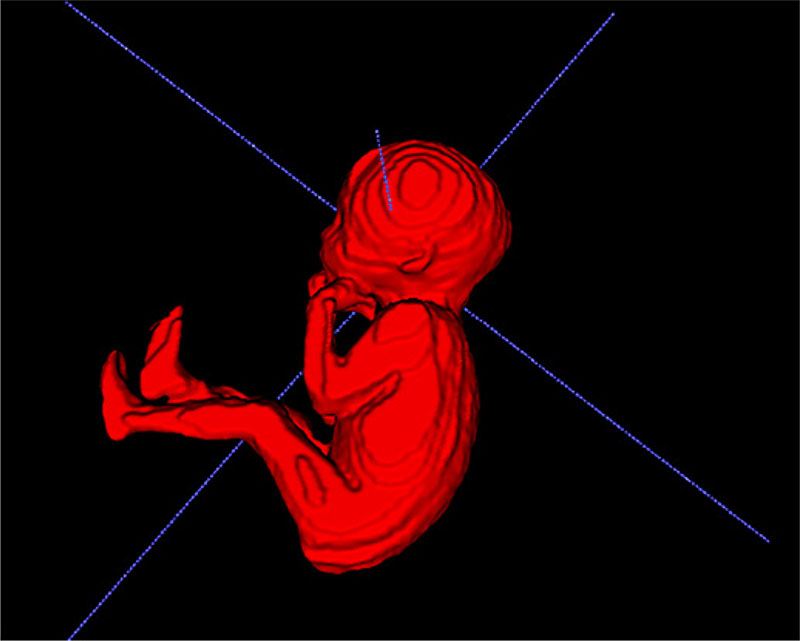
Semiautomatic segmentation of MRI whole fetal body volume.
